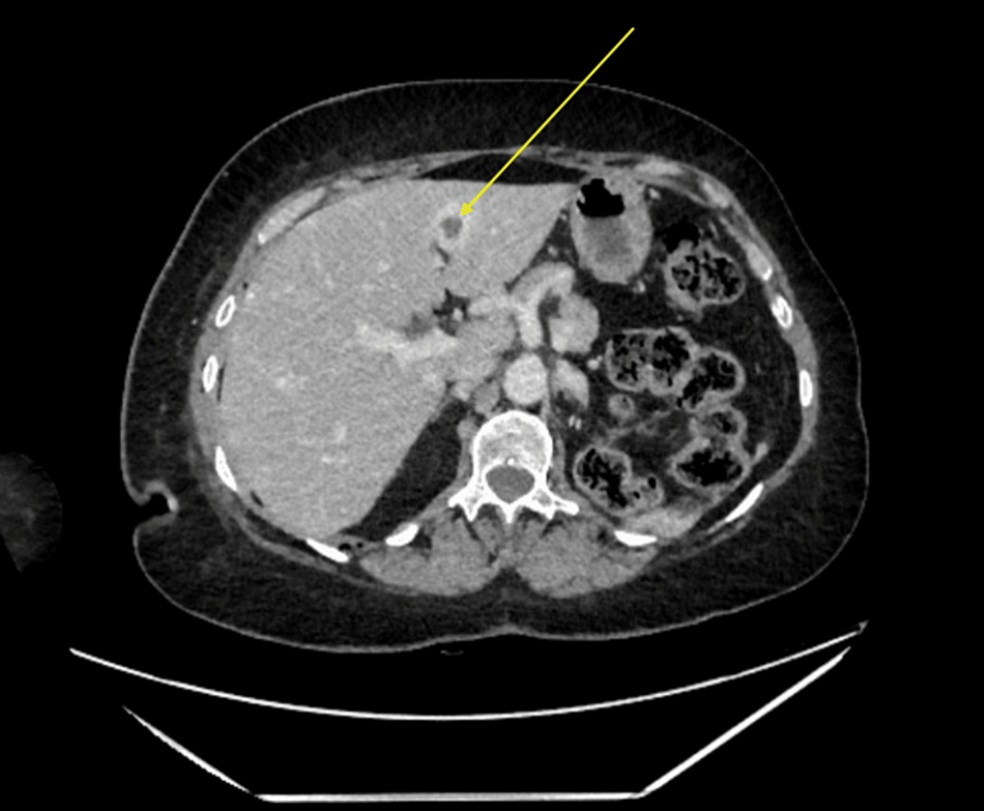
Demonstration of a liver metastasis on a follow-up CT-TAP. CT-TAP: CT of the thorax, abdomen, and pelvis (CT-TAP)
